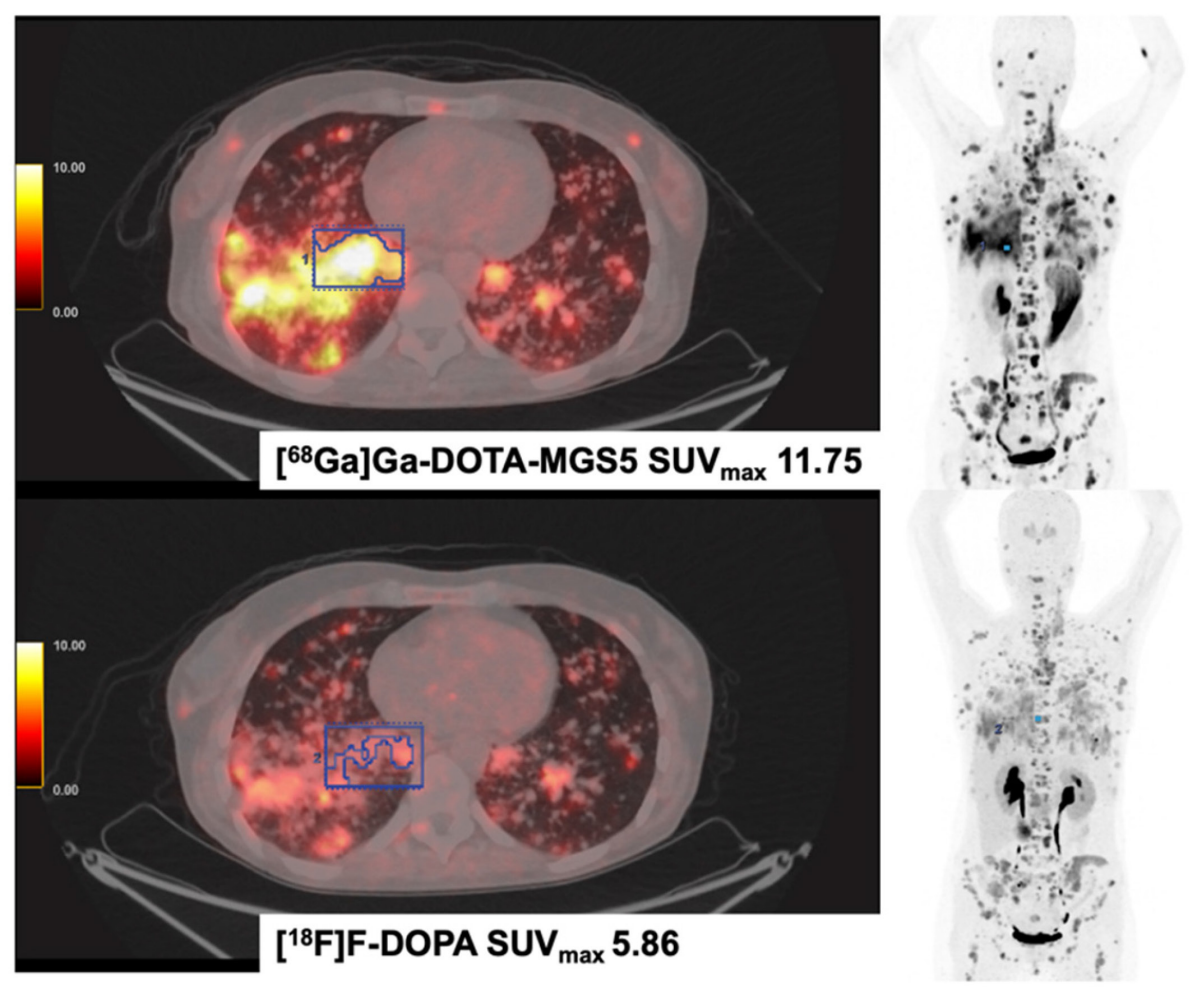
[^68^Ga]Ga-DOTA-MGS5 (DOTA-DGlu-Ala-Tyr-Gly-Trp-(N-Me)Nle-Asp-1-NaI-NH_2_) and [^18^F]F-DOPA PET/CT in a Patient with Medullary Thyroid Cancer (MTC). The patient was diagnosed with medullary thyroid cancer in 2014 (Ki-67 15%). Disease progression was evidenced after treatment with sorafenib, vandetanib, cabozantinib and long-acting octreotide. In 2023, disseminated metastases were seen in both examinations, [^18^F]F-DOPA and [^68^Ga]Ga-MGS5 PET/CT. The patient currently receives treatment with the CCK2-targeting [^177^Lu]Lu-labelled antagonist [^177^Lu]-Lu-PP-F11N (DOTA-(DGlu)6-Ala-Tyr-Gly-Trp-Nle-Asp-Phe-NH2) in Basel, Switzerland.
